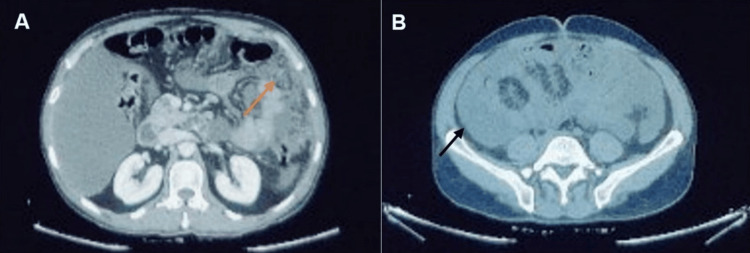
Computed tomography imaging findings obtained at hospital admission Contrast-enhanced computed tomographic axial images showing (A) thickening and nodularity of the peritoneum and omentum (orange arrow) and (B) presence of pelvic ascites (black arrow)
